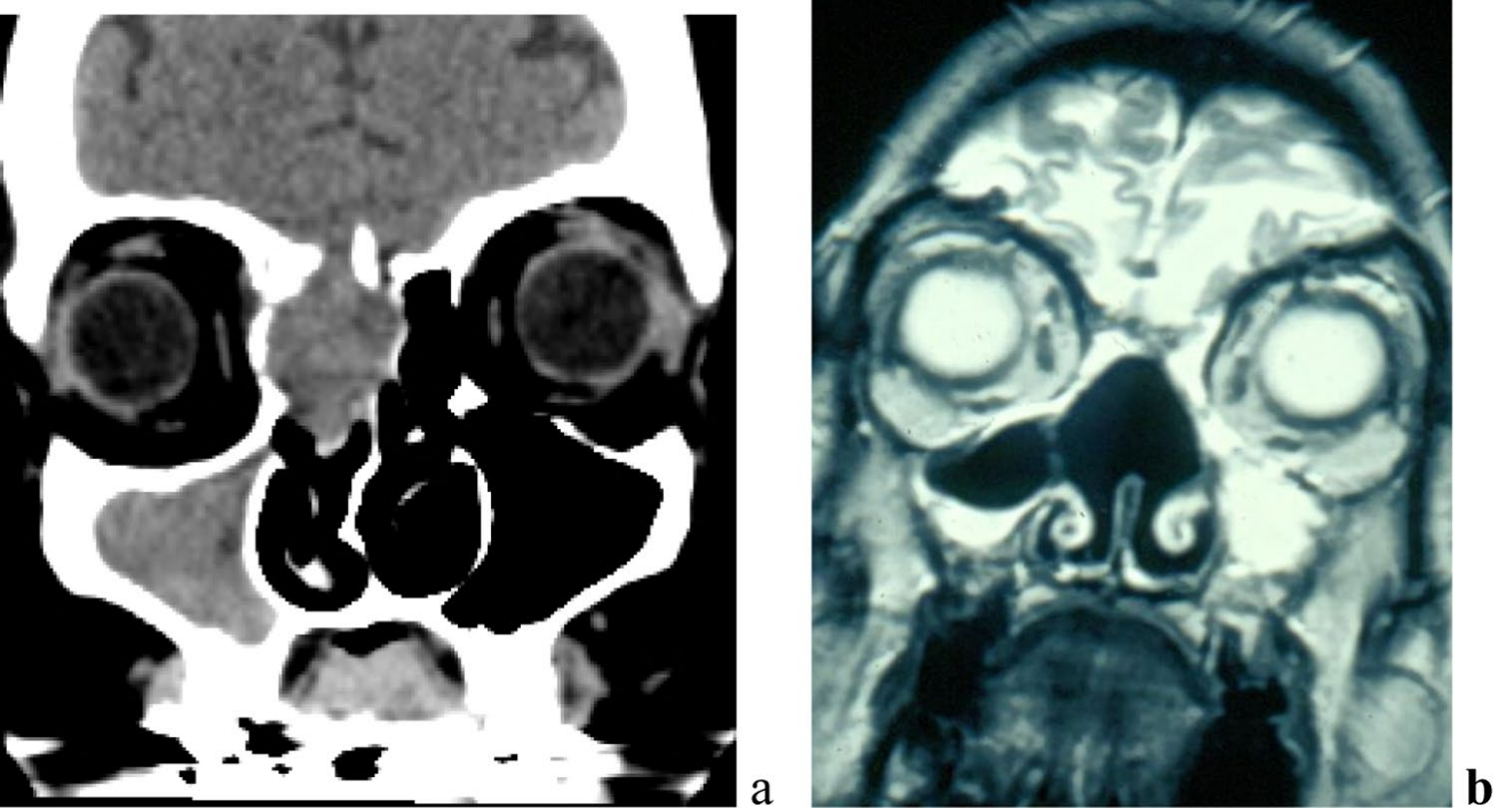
Pre-operative CT scan (a) and postoperative MRI scan (b) (Case 3)
